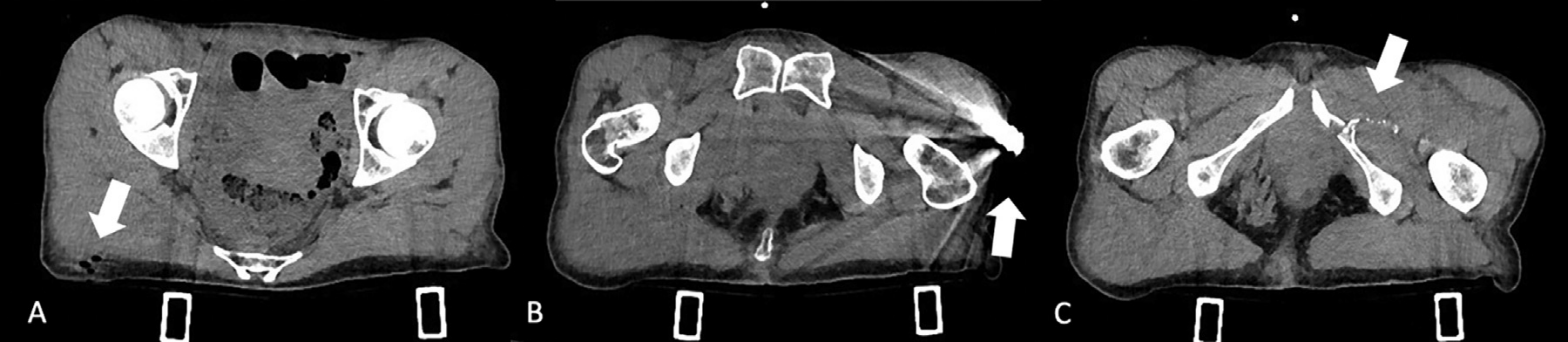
Axial CT scan during the pre-contrast phase showing (A) gunshot entry hole in the right gluteal region, (B) the retained bullet localized in the left coxofemoral subcutaneous region, (C) fragmented fracture of the left ischiopubic branch with evidence of multiple tiny bone pieces retained within the ipsilateral adductor muscles.
